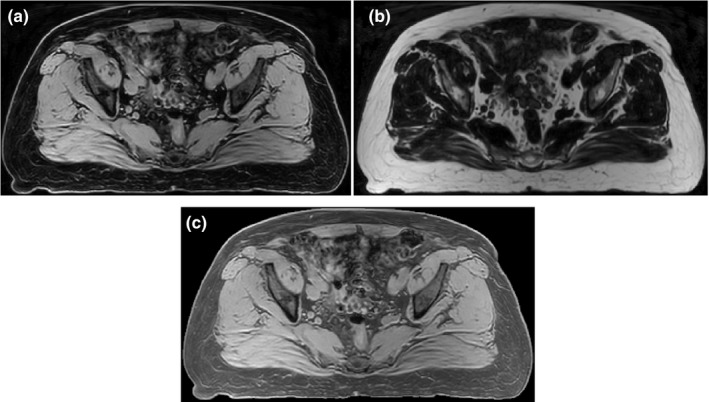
Example of water‐only (a), fat‐only (b), and fat‐enhanced water‐only image (c). As shown in (c), the contrast between fat and air regions was improved in fat‐enhanced water‐only image compared to water‐only image. Water‐only image was used to create the CT‐MR atlas and fat‐enhanced water‐only image was used for atlas propagation.
